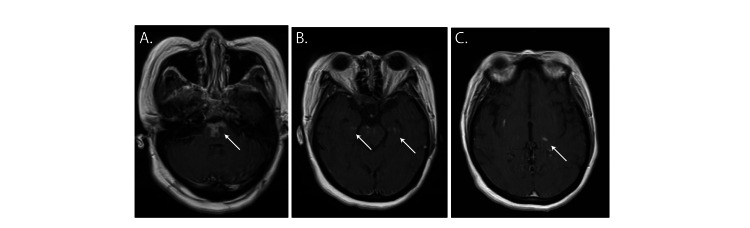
MRI scan two weeks after admission shows contrast enhancement. Axial T1 post-contrast images (A-C) show areas of contrast enhancement in the pons, mesial temporal lobes, and thalami (white arrows).
